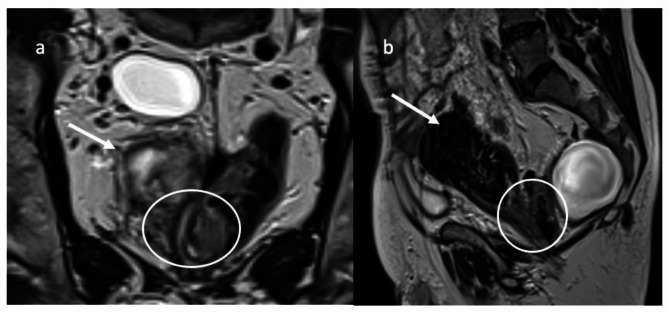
MRI images from the staging. (a) coronal T2, (b) sagittal T2 imaging. The white arrow points at the massively dilated rectal stump with fecal remnants. The white circles point at the anastomotic region
